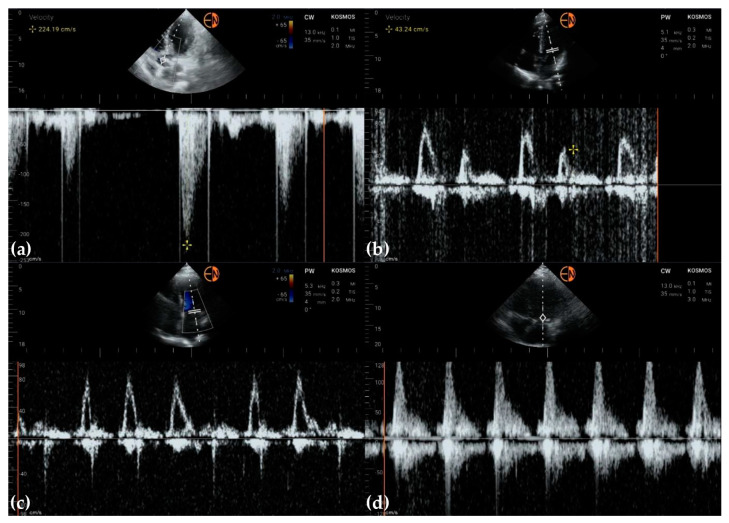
(a) Continuous wave Doppler shows post-extrasystolic aortic velocity; (b) pulsed wave Doppler of mitral flow in a patient with normal diastolic LV function; tissue Doppler imaging is not yet available on any handheld ultrasound, to differentiate from pseudo-normal diastolic function; (c) absence of A waves in a patient with long-standing atrial fibrillation; (d) trans-mitral flow with absent A wave in a man presenting with 2:1 atrial flutter.
